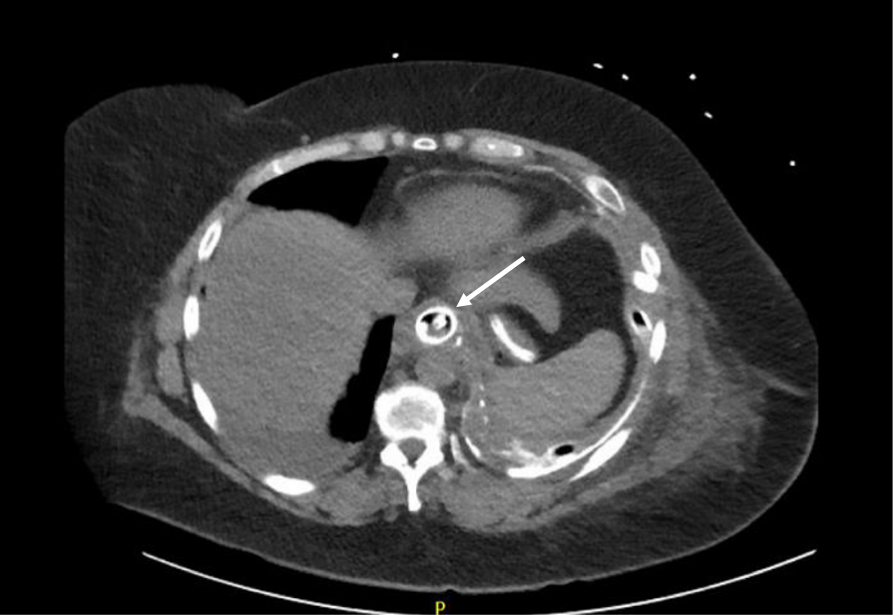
Computed tomography following surgery, showing esophageal stent (*arrow*) and residual contrast in the mediastinum
